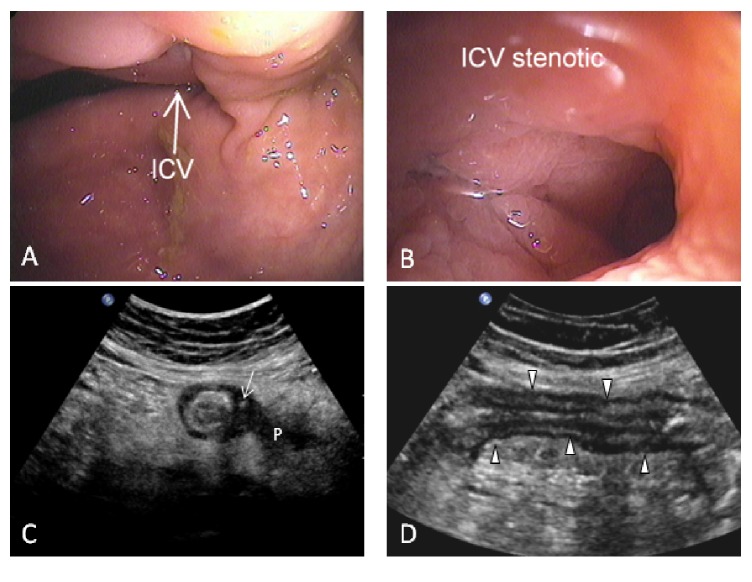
Complicated Crohn's disease on ultrasound only. 45-year-old female with a 2-year history of Crohn's disease. Colonoscopy failed to intubate the terminal ileum and did not detect any active disease. (A) shows the ileocecal valve (ICV) and (B) shows a close-up view of the orifice with limited view of the terminal ileum (TI). (C) An axial ultrasound image of the TI shows mural thickening and surrounding inflammatory fat. The wall is eccentrically thickened and shows intramural extraluminal air (arrow), suggestive of localised perforation. A poorly marginated hypoechoic zone within the inflammatory fat suggests a phlegmon (P). (D) is the corresponding sagittal image of the thickened TI. Interpretation: acute local perforation and phlegmon. After ultrasound, the patient was referred to surgery.
